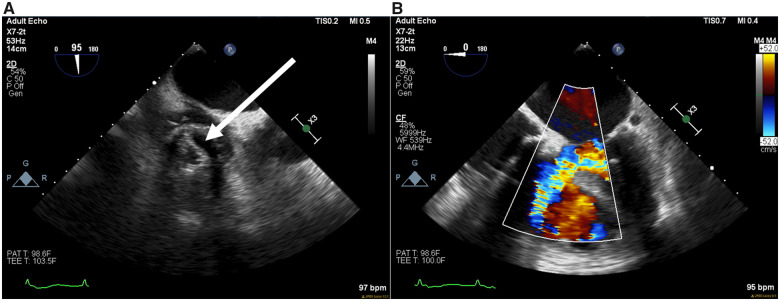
Transesophageal echocardiography. (*A*) Short-axis view highlighting the immobile mechanical septal leaflet (arrow) of the tricuspid mechanical prosthesis. (*B*) Iatrogenic ventricular septal defect is shown with left to right shunting on colour flow Doppler.
